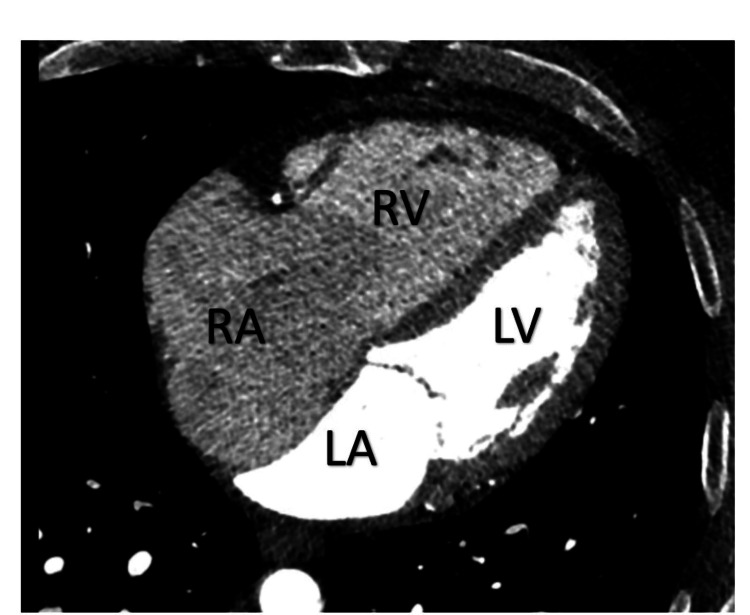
Computed tomography (CT) of heart in four-chamber view shows marked enlargement of the RA and RV. RA: right atrium, RV: right ventricle, LA: left atrium, LV: left ventricle.
